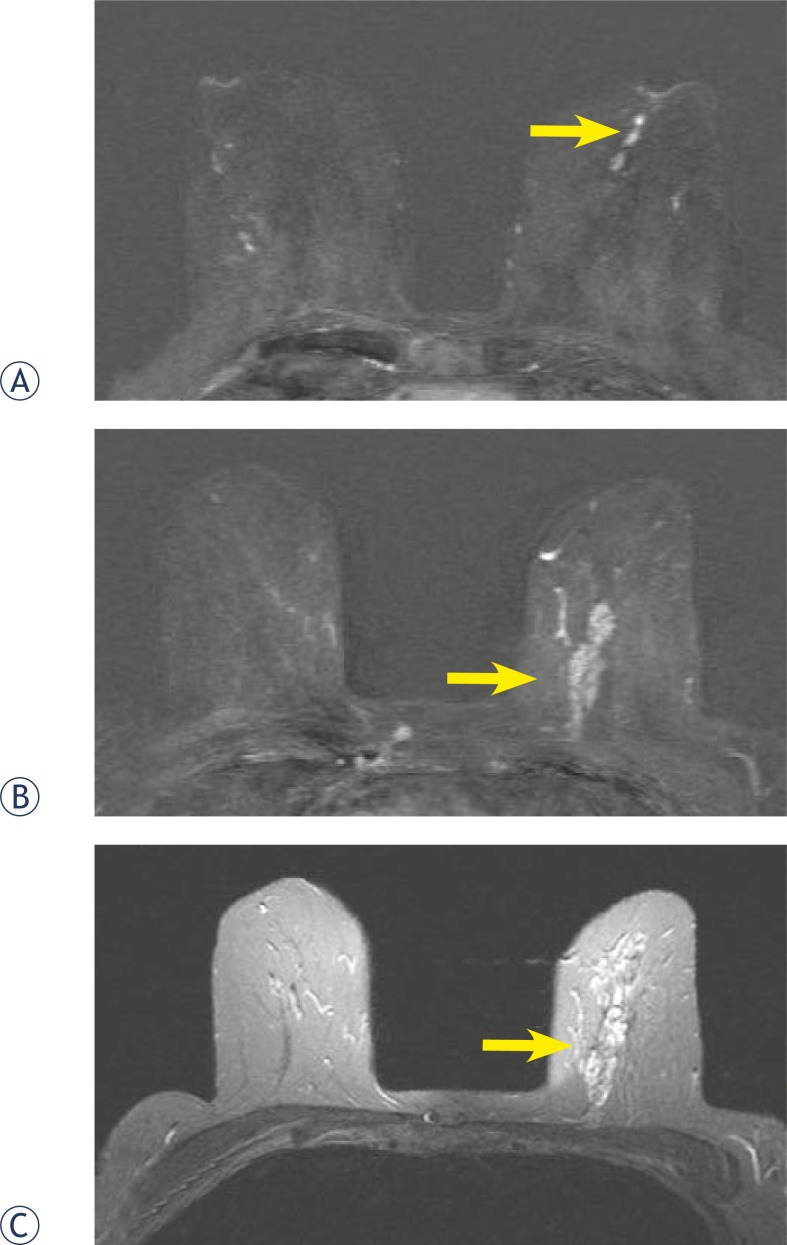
65 year old woman with a history of nipple-discharge in her left breast. Mammographic asimmetry. Ultrasound images showed dilated empty ducts, ductography was suspicious for papilloma. Axial T1-weighted subtracted image after Gadolinium injection (2nd minute) .(a) small round sharply circumscribed masses within a duct sistem–papillomas.(b) segmental, clumped, asymmetric enhancement, fast initial increase and postinitial wash out. (c) high signal intensity on T2–weighted image - BI-RADS 5. Pathologic diagnosis through MRI-guided vacuum-assisted biopsy was massive DCIS with foci of microinvasion.
